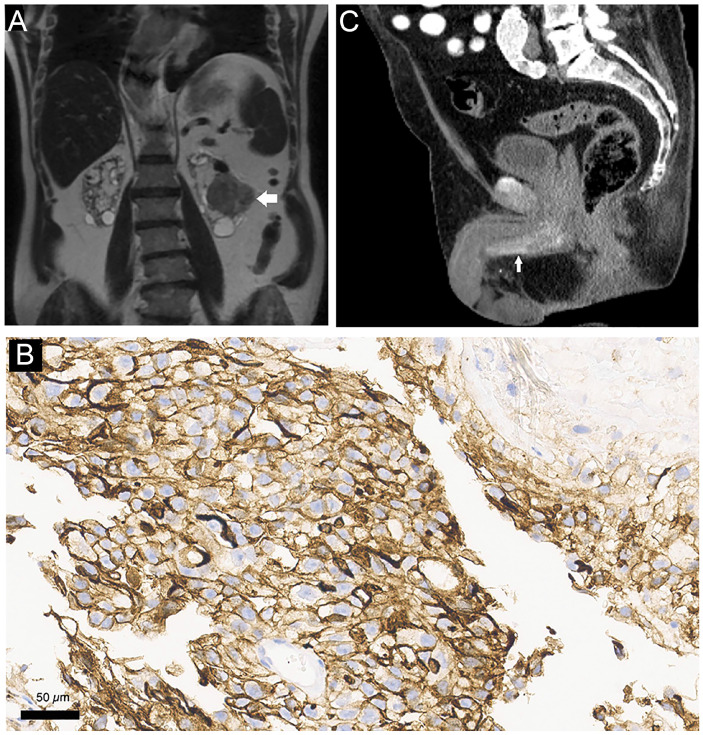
(A-C) – MRI (A: coronal T2WI) demonstrates a solid renal lesion (horizontal arrow in A) in the left kidney, which was proved to represent renal cell carcinoma. The lesion has irregular contours and heterogeneous signal intensity in T2WI. Polycystic kidney disease is also evident with multiple bilateral renal cysts. Pathological analysis of an endoscopically resected urethral lesion confirmed metastasis from RCC. The tumor cells are CD10 positive (B: CD10, x400). A CT performed two months after resection (C: sagittal image after intravenous contrast injection, arterial phase) shows early enhancement (vertical arrow in C) Along the penile bulb and proximal body of the penis, raising suspicion for regrowth of the metastatic lesion.
